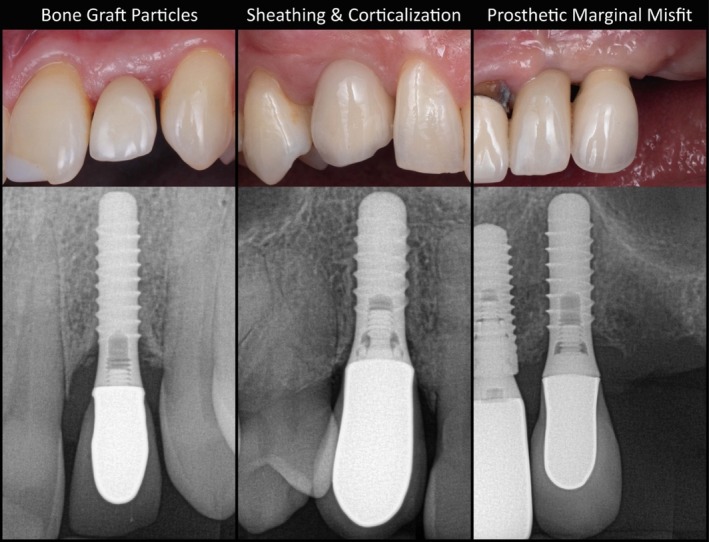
Clinical images and corresponding periapical radiographs of representative cases with adverse radiographic findings.
